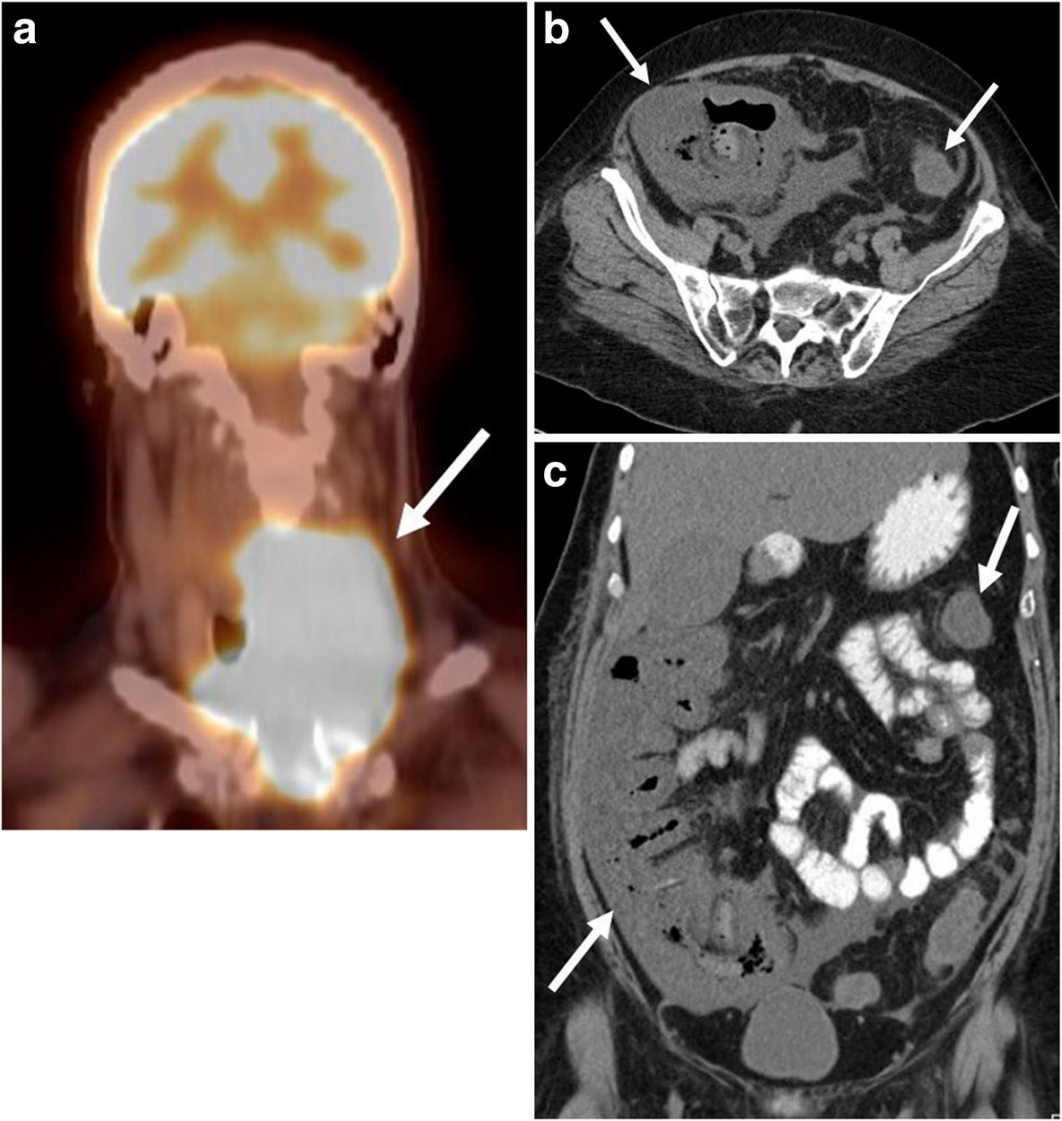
Treatment-related neutropenic colitis. A 58-year-old male initially presenting with a left neck mass. a Coronal fused PET-CT demonstrates a large, hypermetabolic left neck mass (arrow) causing tracheal deviation. The mass was subsequently biopsied as Burkitt lymphoma, and the patient was subsequently started on hyper-CVAD therapy. After cycle #3, the patient was admitted for abdominal pain and neutropenic fever. b Axial and (c) coronal non-contrast CT images obtained in the emergency department show diffuse colonic wall thickening and inflammatory stranding (arrows). Findings are consistent with treatment-related colitis
